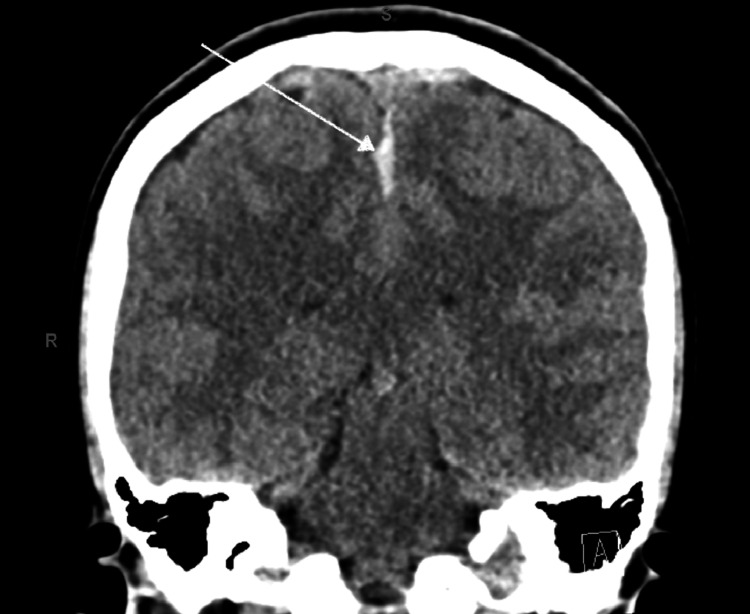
CT head plan coronal view revealing subdural hematoma along the midline falx (white arrow). CT: computed tomography
